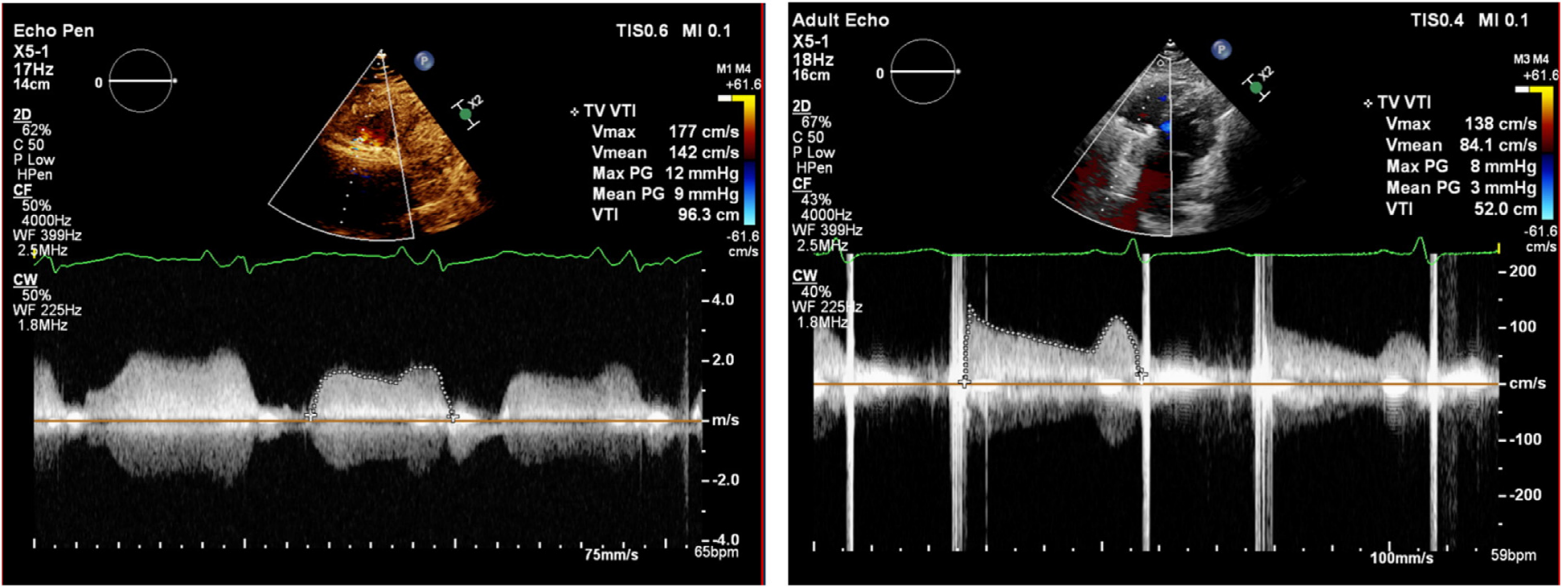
Pulsed Wave Doppler Across Mechanical Tricuspid Valve Replacement Pre-thrombolysis (left) and post-thrombolysis (right).
